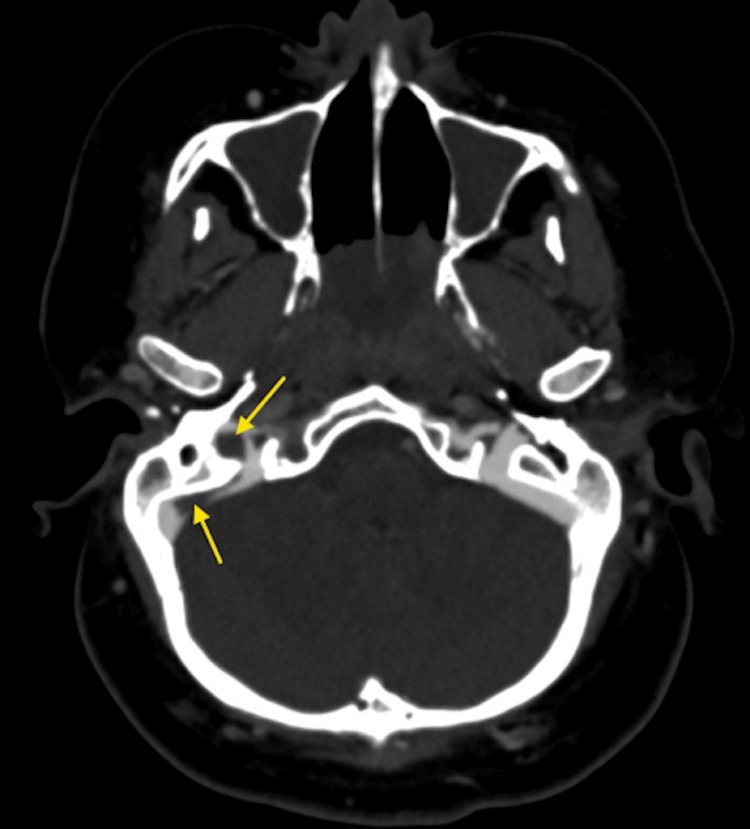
CT Head Venography (axial) demonstrates large non-occlusive thrombus in the right sigmoid sinus extending to the jugular bulb (arrows). No interval progression compared with baseline, indicating stable thrombosis under anticoagulation.
